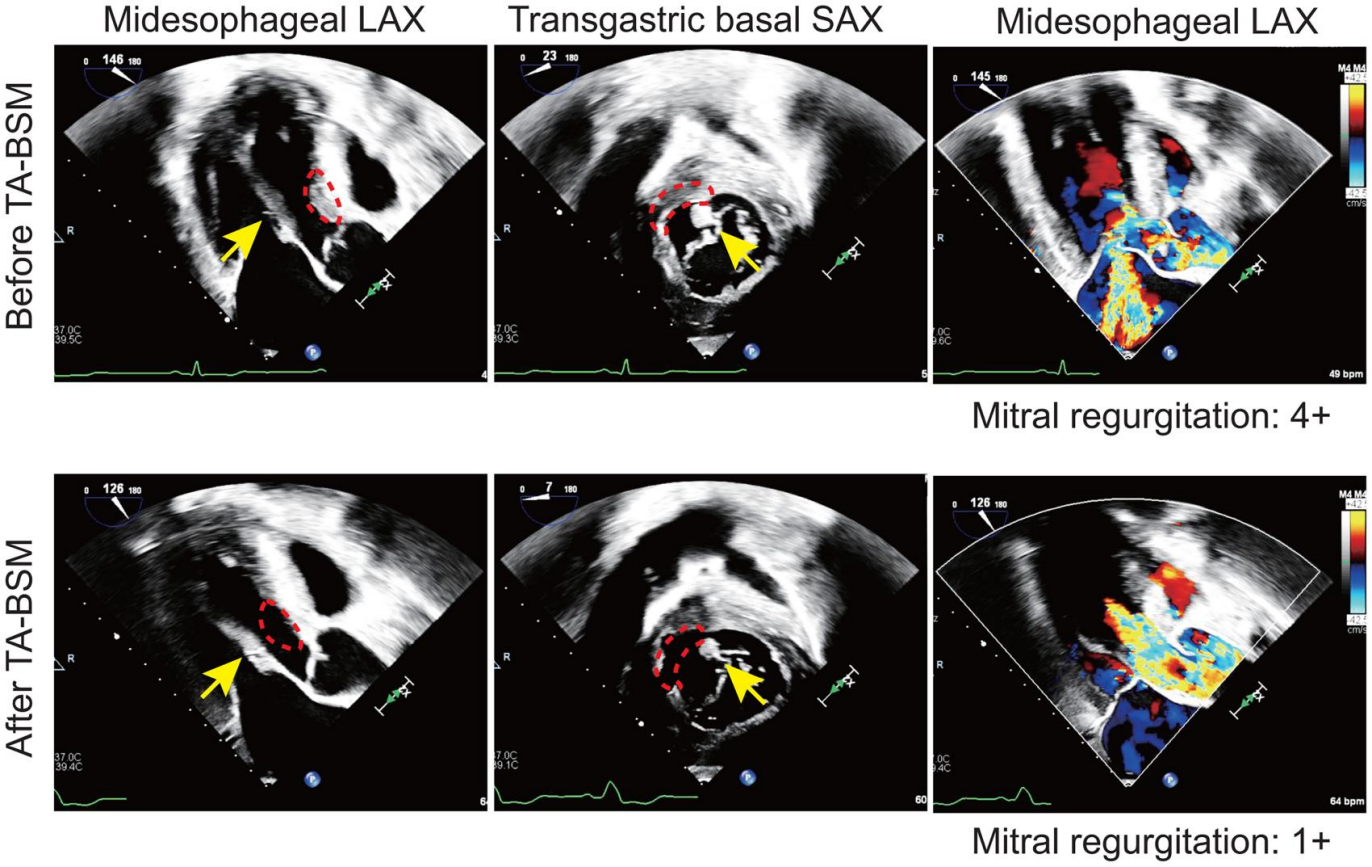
Representative Transoesophageal Echocardiographic Image During Transapical Beating-Heart Septal Myectomy. The echocardiographic images showing the long-axis (LAX) and short-axis (SAX) views of transoesophageal echocardiography before (top) and after (bottom) TA-BSM. The resected zones are marked by the red dotted curve and the yellow arrows identify anomalous insertion of PMs into the mitral apparatus
